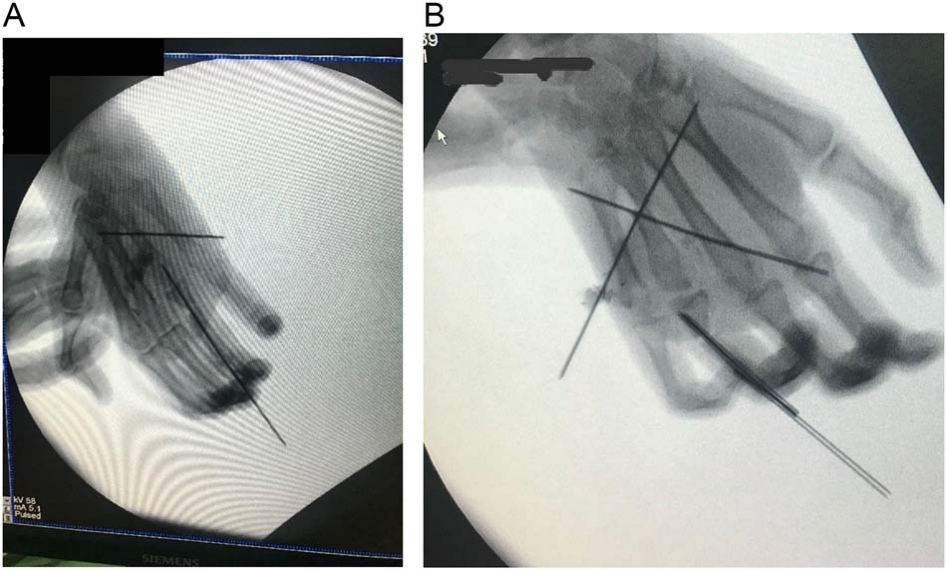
(A) Intraoperative X-ray showing K-wire inserted through the interphalangeal joint crossing the bone flap reaching up to the second metacarpal and another K wire inserted through the interphalangeal joints. (B) After fixation.
